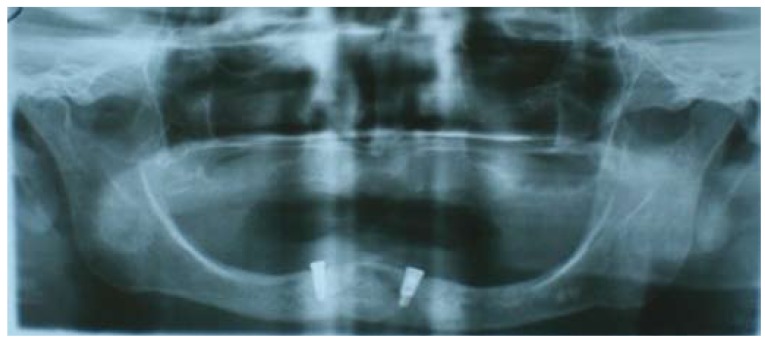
Orthopantomogram. Severe mandibular atrophy and 2 osseointegrated implants in the intermentonian region.
